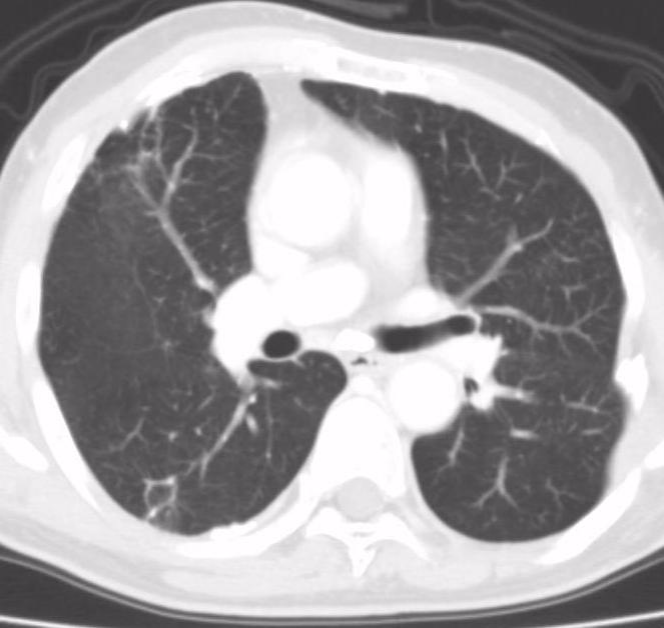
CT images before SBRT (Pt. No. 8).
